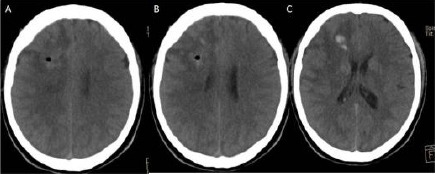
A, B, C: Cranial CT following the procedure showing little amount of hemorrhage that is not caused any symptoms and signs*.
